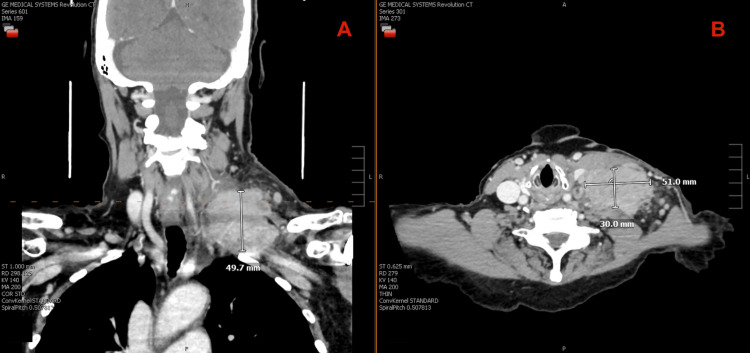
Contrast-enhanced CT of the neck obtained September 30, 2024, approximately two weeks after ultrasound-guided core needle biopsy. Coronal (A) and axial (B) views demonstrate enlargement of the same mass to 49 × 30 mm with mild displacement of adjacent vasculature, presumed to represent a hematoma.
